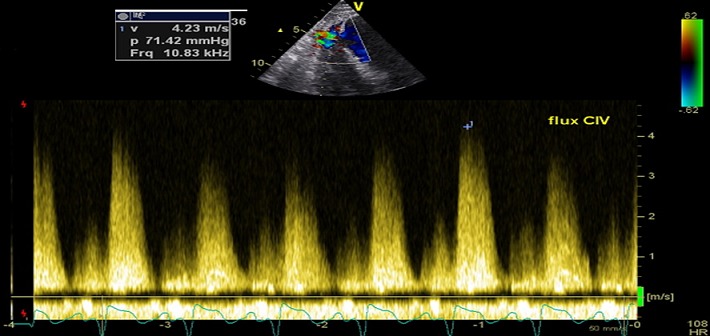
Aspect du flux de la CIV au Doppler continu
